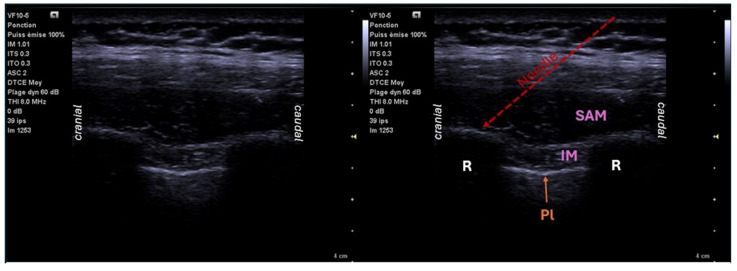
Ultrasound location for serratus block: sagittal view T4–T5. R: rib; Pl: pleura; IM: intercostal muscle; SAM: serratus anterior muscle.
